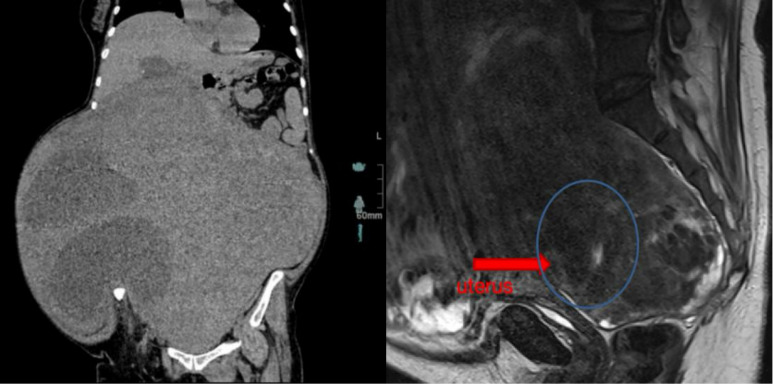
Abdomen and pelvis (a) CT. (b) MRI.
